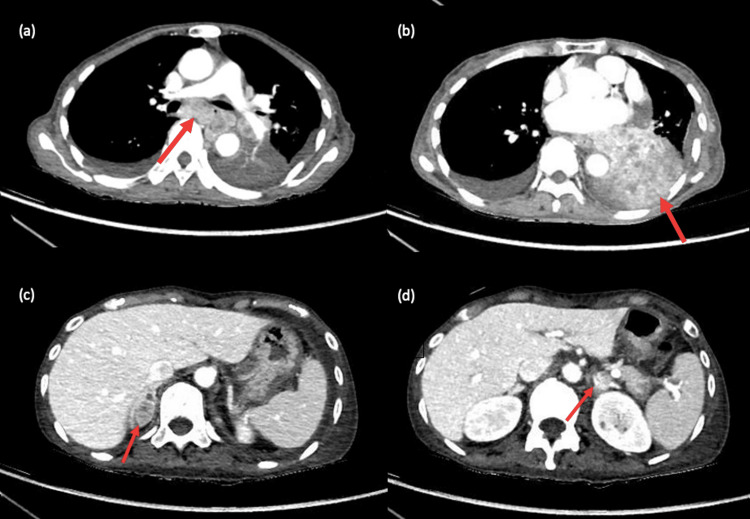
CT thorax, abdomen, and pelvis. (a) Subcarinal lymphadenopathy, (b) necrotic left lower lobe lung mass, (c) right adrenal metastatic nodule, and (d) left adrenal metastatic nodule. CT, computed tomography
